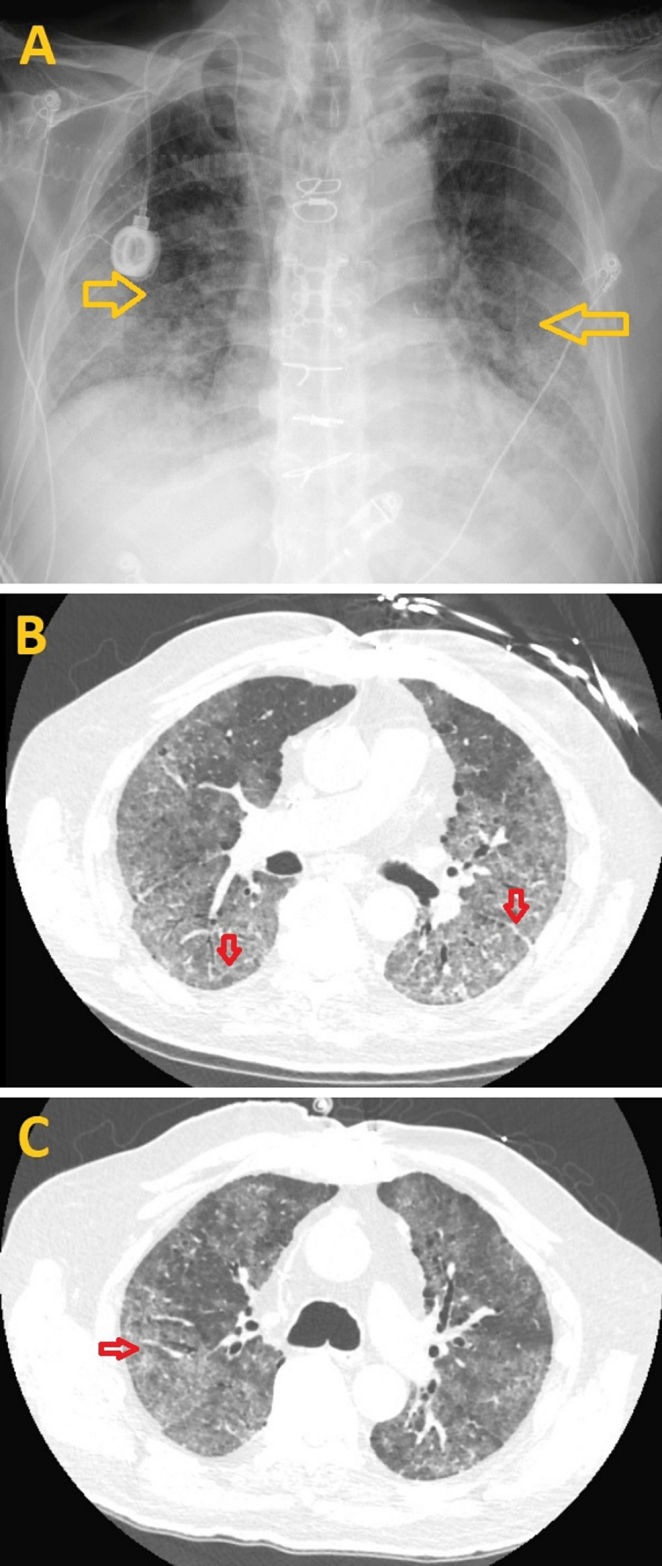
Repeat chest X-ray (CXR) and high-resolution chest CT scan findings 6A: CXR shows increased bilateral interstitial opacities involving the mid and lower zones of the lungs (yellow arrows). 6B, 6C: A high-resolution chest CT without contrast demonstrates stable bilateral ground glass opacities with septal thickening (red arrows).
